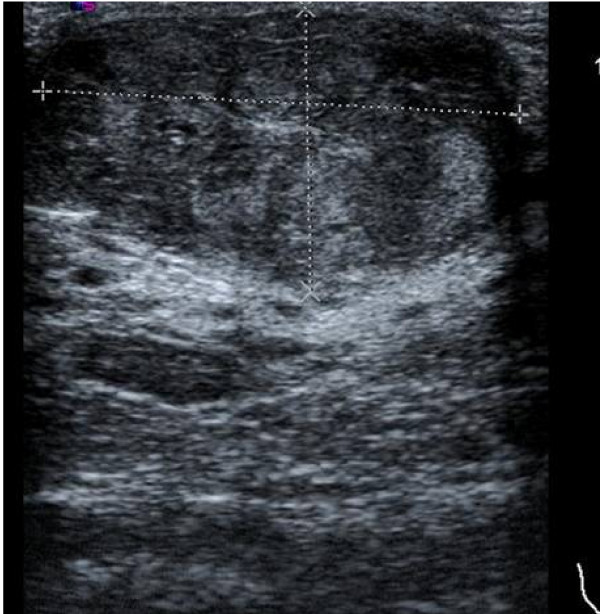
Ultrasound evaluation of the lesion.
